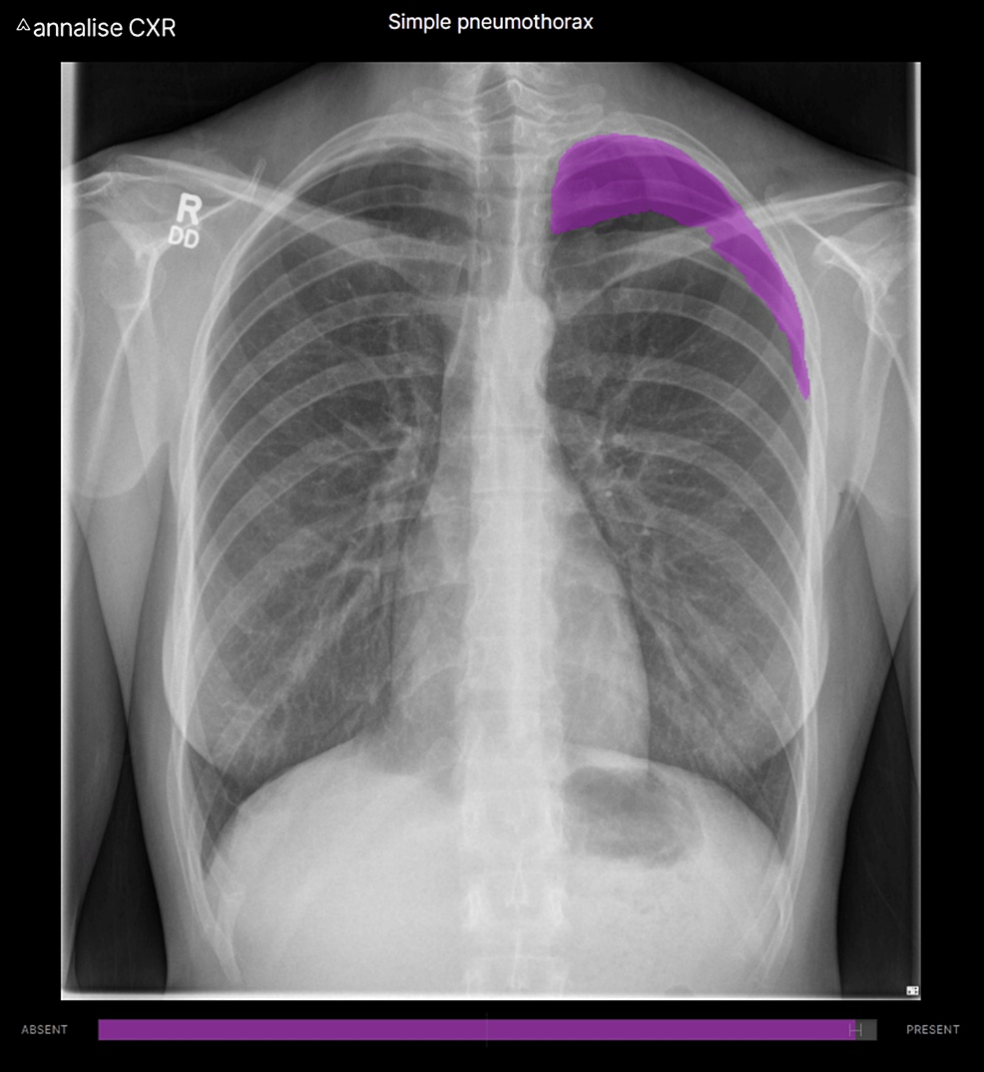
This patient’s chest radiograph displayed within Annalise Enterprise CXR (Annalise; Sydney, Australia), with AI-identified finding of pneumothorax overlaid.
